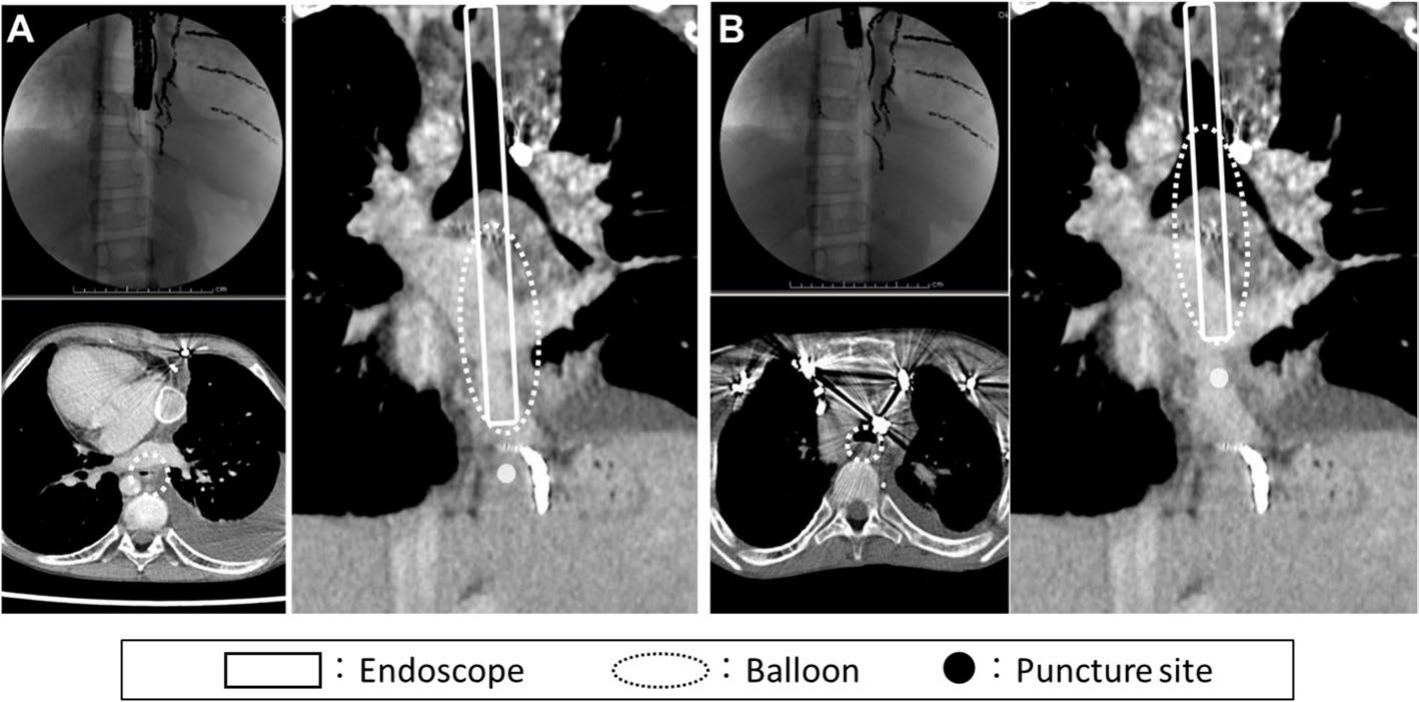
Intraesophageal balloon position inferred from perioperative CT and fluoroscopic images during injection of sclerotic agents into the varicose vein of the gastric cardia (A) and of the esophagus (B). Intraoperative fluoroscopic image (upper left), perioperative axial (lower left), and coronal (right) CT images. Note the balloon located behind the common pulmonary venous cavity (A) and the coils in front of the tracheal bifurcation (B)
